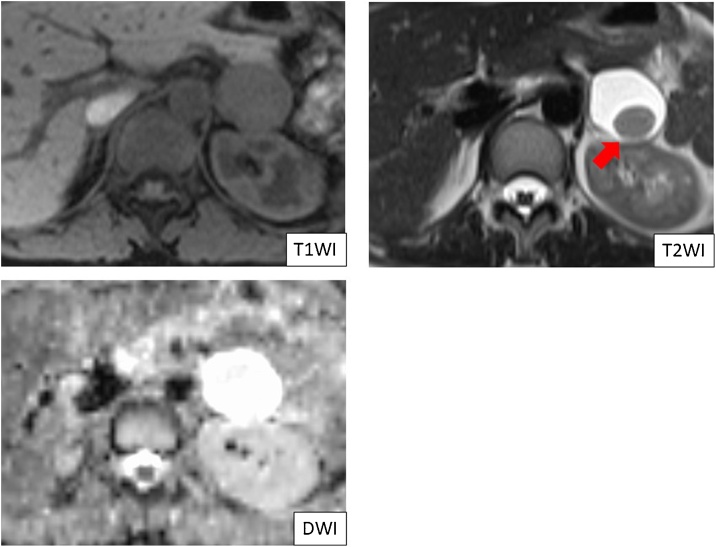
Magnetic resonance imaging. The cystic lesion shows low intensity in T1-weighted images, high intensity in T2-weighted images, and high intensity in diffusion-weighted images. In T2-weighted images, a round-shaped low intensity area, measuring 11 mm, was detected in the cystic lesion.
